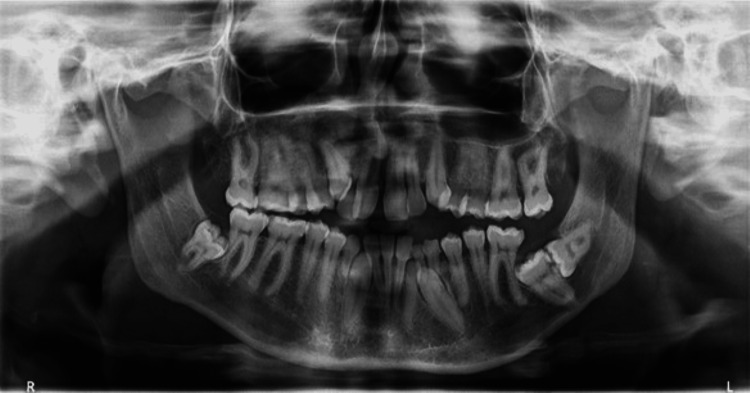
Patient's pretreatment panoramic radiography
